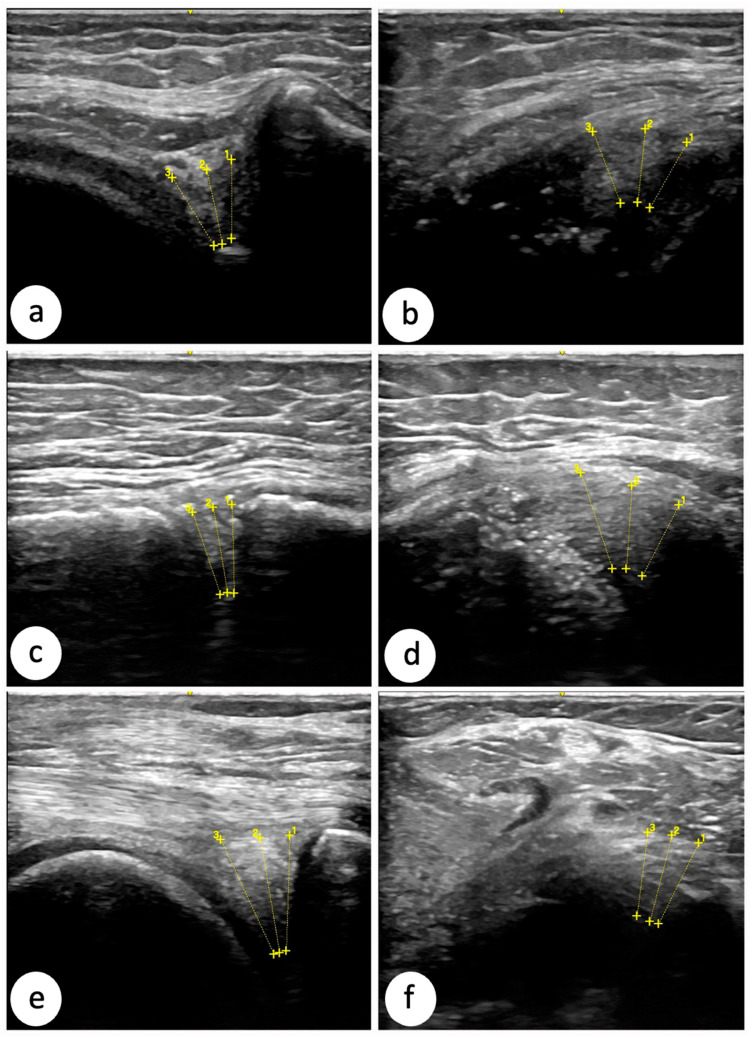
Longitudinal and transverse ultrasound scans of each meniscus region with examples of ultrasound measurements. (a) The transverse section of the anterior region of the meniscus; (b) longitudinal section of the anterior region of the meniscus; (c) transverse section of the middle region of the meniscus; (d) longitudinal section of the middle region of the meniscus; (e) transverse section of the posterior region of the meniscus; (f) longitudinal section of the posterior region of the meniscus.
